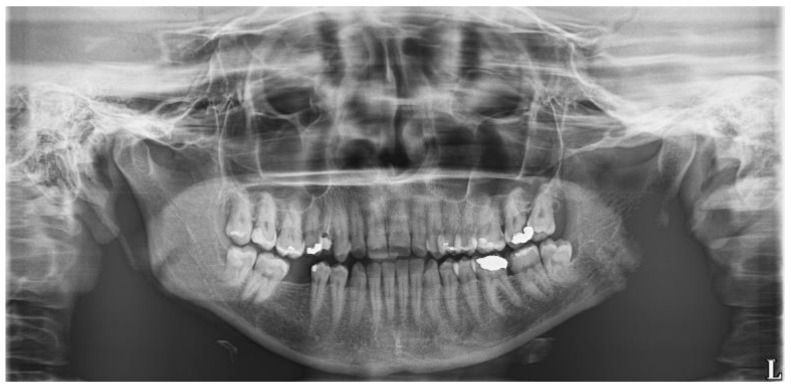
The orthopantomogram of the patient 2. Tooth No. 38 is in normal position, slightly distally inclined, with a semilunar burnout distally. The OPG was not taken at our department.
